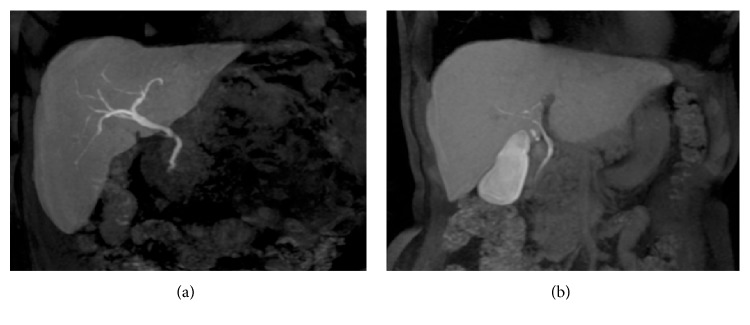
Gadolinium-enhanced MRC. MIP images of 3D T1-weighted spoiled gradient echo. (a) shows opacification of biliary tract after Gd-EOB-DTPA administration; hepatobiliary phase could be obtained 20 minutes after intravenous contrast. In a different patient, (b) demonstrates excretory phase obtained after gadobenate dimeglumine intravenous administration. In this case, the elimination of contrast media is provided by the kidney (95–97%) and the biliary system (2–5%): a satisfactory opacification of the biliary tree—in patients with preserved biliary function—is observed at least 60 minutes after contrast administration.
